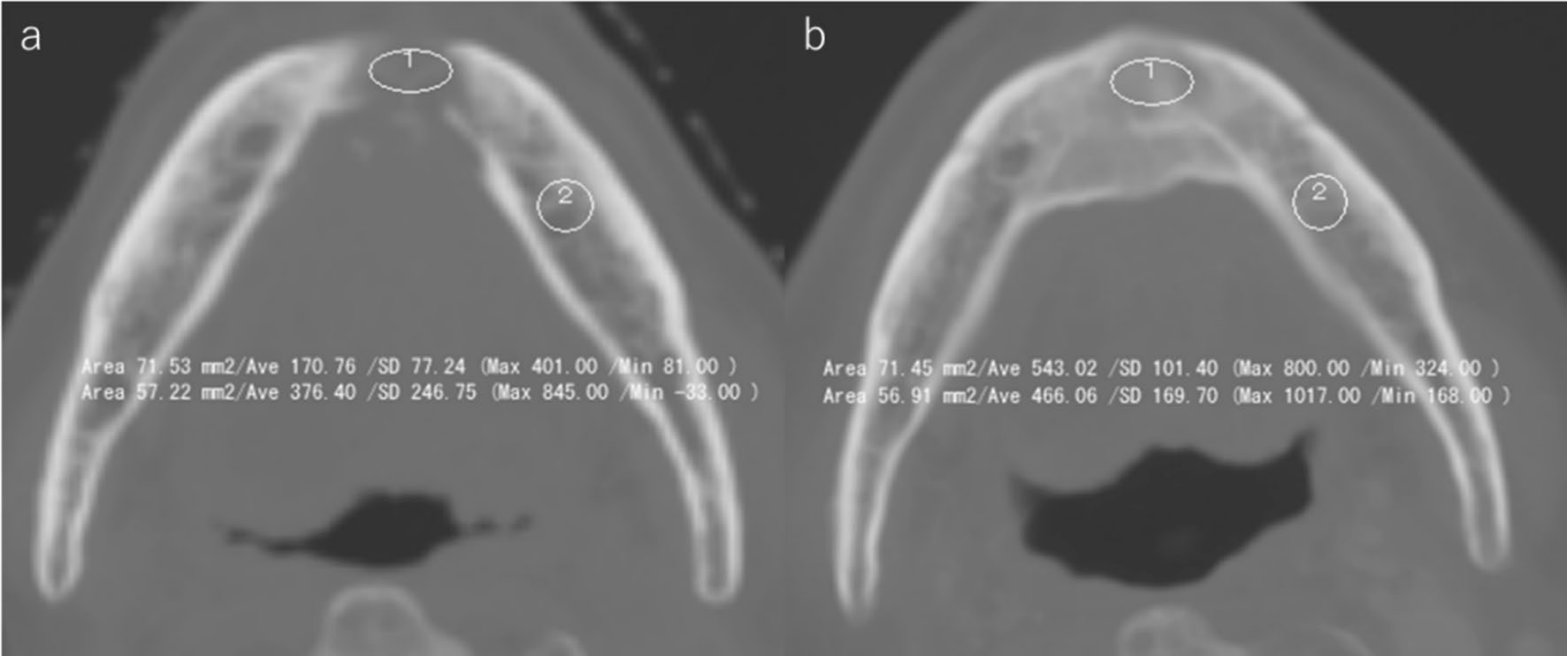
CT images at bone destruction a and remineralization b, with regions of interest (ROIs) placed for CT value measurement. ROIs were set within the trabecular bone at the destruction site and at the contralateral site or a molar region as reference. Follow-up images were selected at approximately the same level, with ROIs matched in size and location to those at baseline
